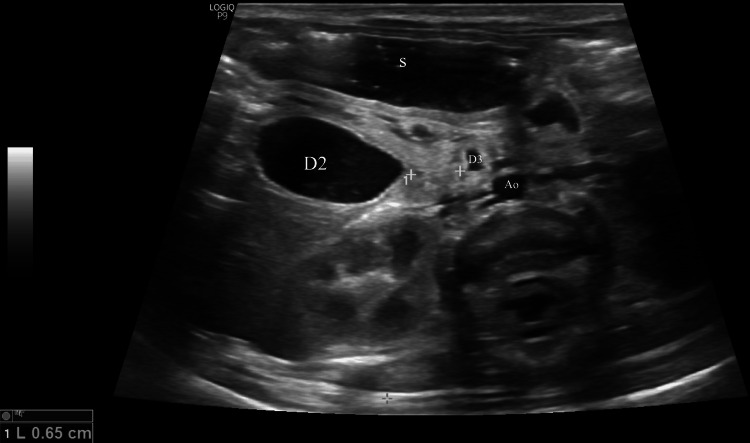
Saline-aided ultrasound image revealing encircled and compressed proximal part of the horizontal duodenum The gray-scale oblique plane demonstrates a dilated prestenotic descending duodenum with a stenotic cone-shaped distal end encircled by pancreatic tissue. The lumen of the horizontal duodenum appears completely obstructed over a 0.65cm segment (between calipers) laterally to the aorta, whereas a normal segment is identified distal to the constricted one, supporting the diagnosis of complete annular pancreas. Ao, Aorta; D2, Descending duodenum; D3, Horizontal duodenum; S, Stomach
